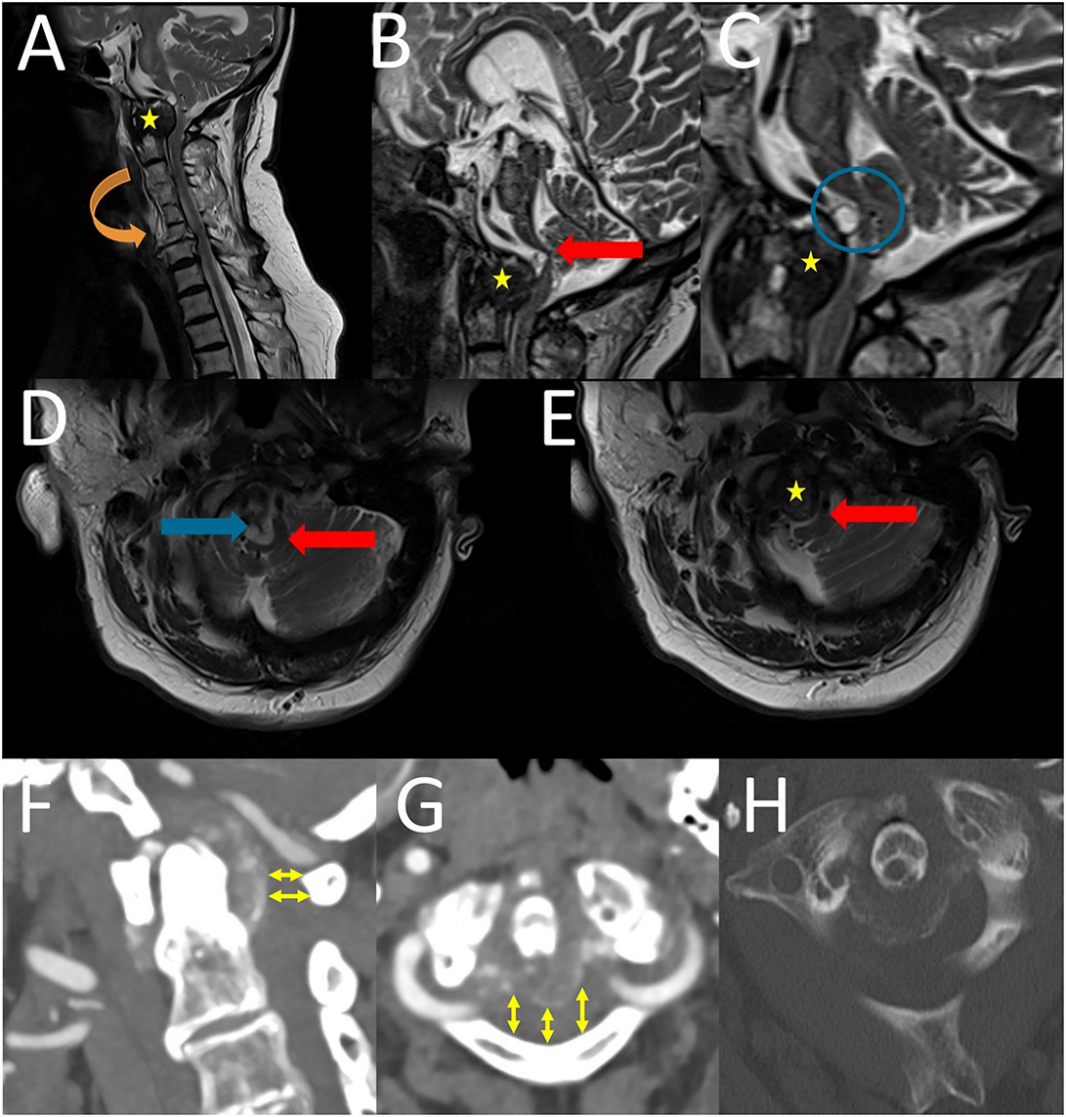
Preoperative sagittal (A–C) and axial (D,E) T2-weigthed magnetic resonance images revealed a lesion of the OP and the retro-odontoid region (yellow stars) with brainstem compression and displacement (red block arrows) and also cystic component (blue block arrows and blue circle). Furthermore, the cervical vertebral bodies C3 to C6 were fused due to Saethre-Chotzen syndrome (orange block arrow). Preoperative CT and CTA of cervical spine (F–H): A sagittal (F) and an axial (G) CTA (soft tissue window) revealed a “normal” anatomy of the vertebral artery and furthermore a spinal stenosis in the level of C 1 (yelow arrows). An axial CT (bone window) showed a “horseshoe” or “crown-like” calcification, which is located posterior to the OP (H).
